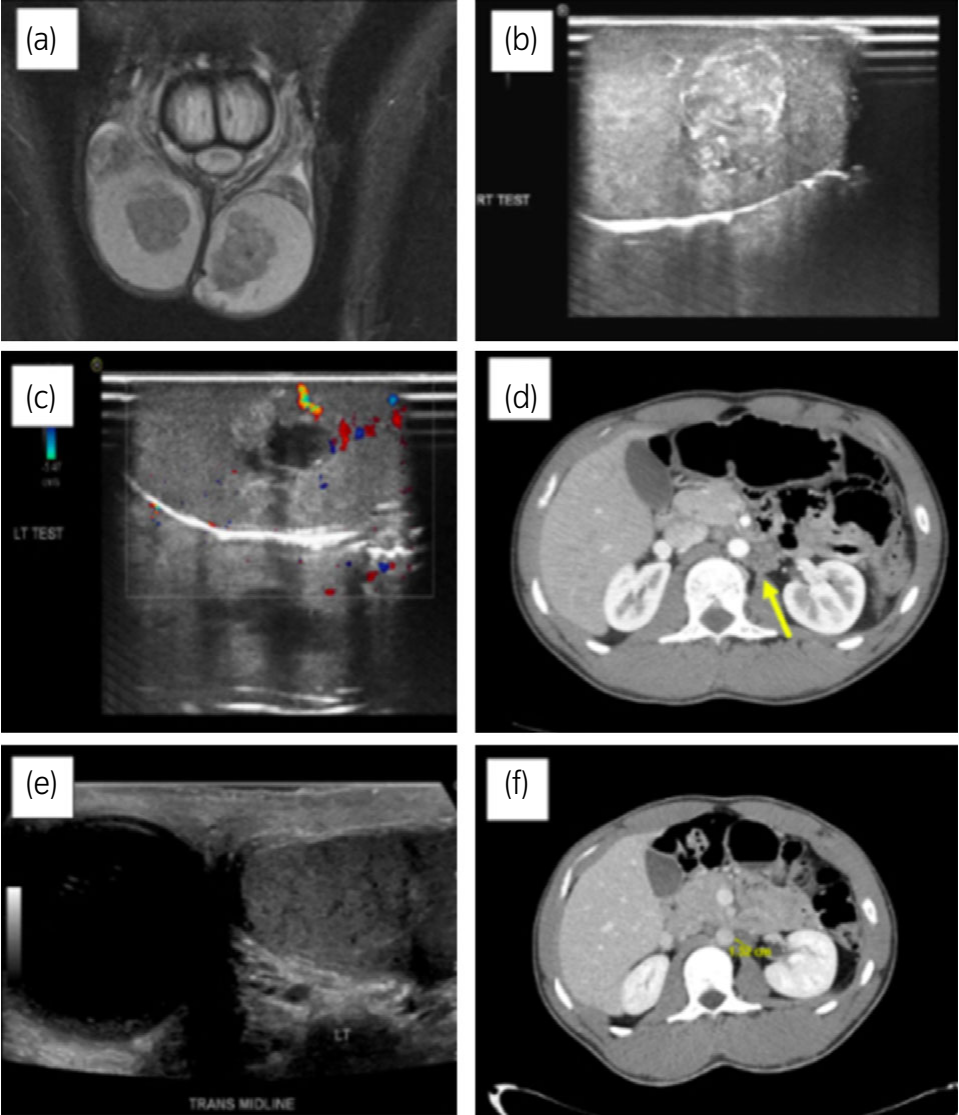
Perioperative and intraoperative imaging. Bilateral testicular masses on preoperative T1‐weighted MRI (a). Intraoperative ultrasound images of right (b) and left (c) testicles. Left para‐aortic soft tissue mass (yellow arrow) identified on CT abdomen at the time of diagnosis (d). Radiographic studies at 2 years of follow‐up demonstrate a right testicular prosthesis and a left testicle without recurrent mass on scrotal ultrasonography (e), as well as the stable appearance of a left para‐aortic soft tissue mass on CT abdomen (f).
